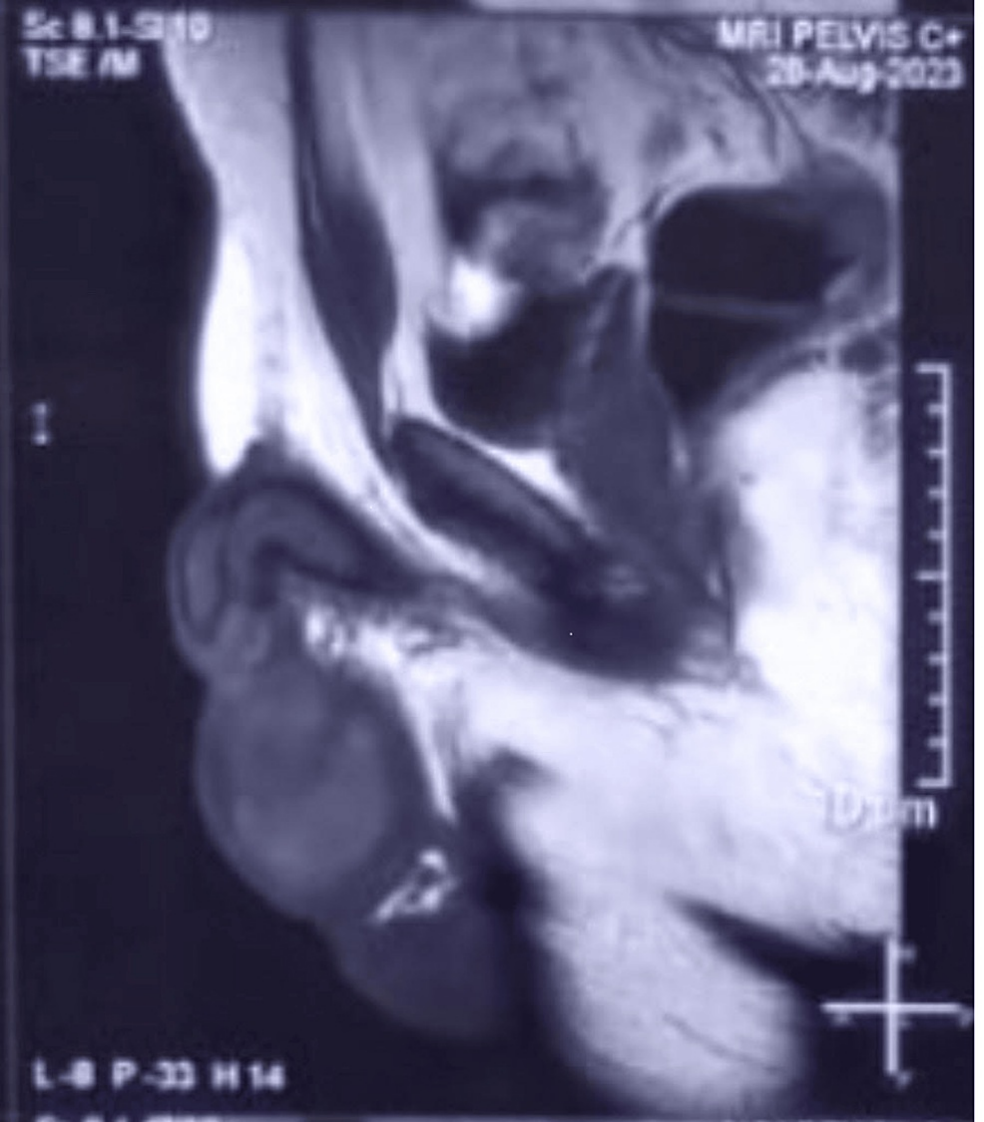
On MRI, a mass was visualized on the upper pole of the right testicle
